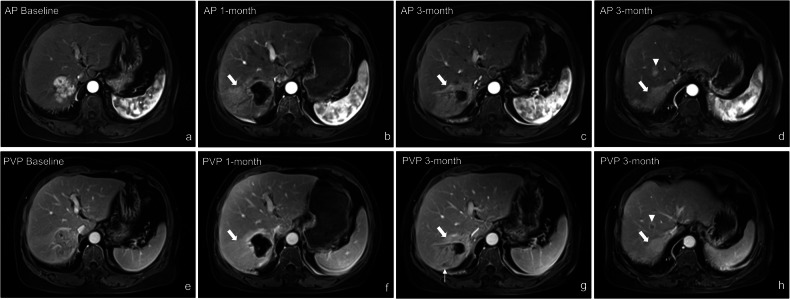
Imaging progression showing the association between THID, biliary injury, and intrahepatic metastasis in a 58-year-old male patient with HCC treated with drug-eluting bead transarterial chemoembolization (DEB-TACE). a, e Baseline axial T1-weighted images in the arterial phase (AP) (a) and portal venous phase (PVP) (e) show a HCC lesion in Segment 7. b, f One-month follow-up MRI reveals the development of THID in Segment 7 associated with an arterioportal shunt (APS) (arrow) visible in both the arterial phase (b) and portal venous phase (f). c, d, g, h Three-month follow-up MRI demonstrates subsequent complications, with biliary injury (straight arrow) visible in the portal venous phase (g) and an intrahepatic metastatic lesion (arrowhead) identified in Segment 7 on both the arterial phase (d) and portal venous phase (h)
